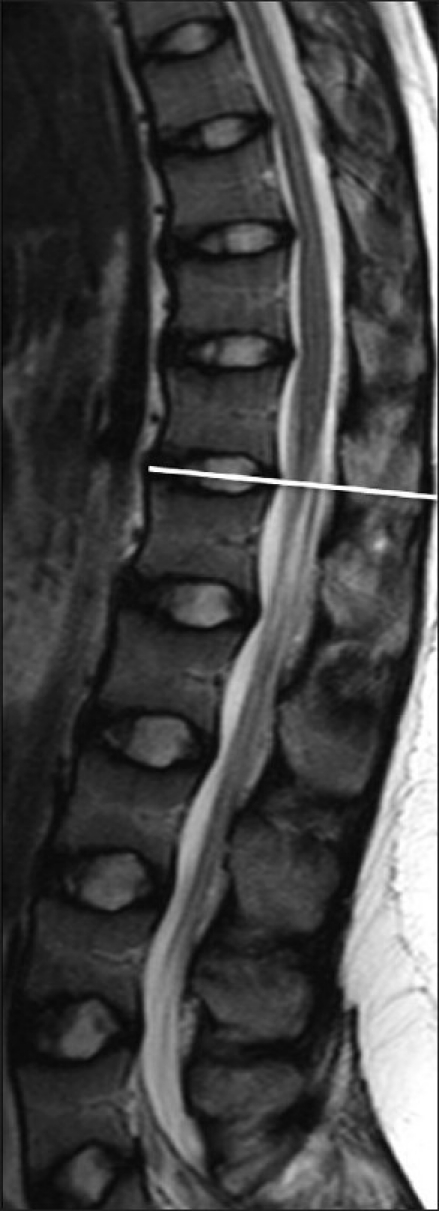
Sagittal T2 image of MRI in an achondroplasia patient comparing with axial image. It showed lowest level of spinal cord (conus medullaris) at T12-L1 disc
